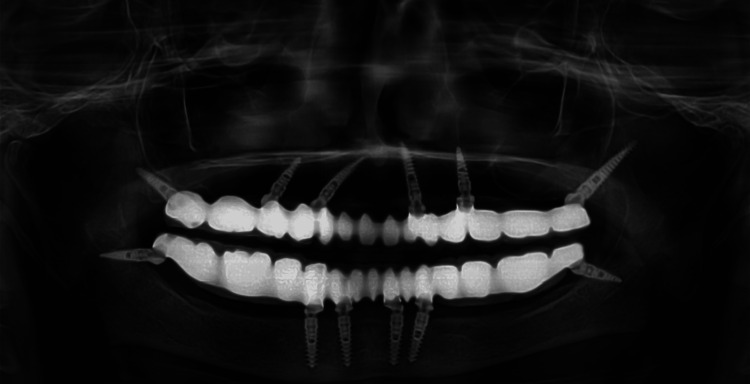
Orthopantomogram of patient at three-year follow-up
